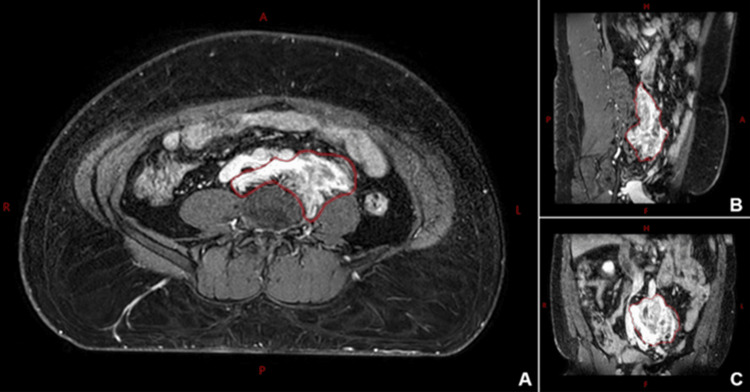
Retroperitoneal ganglioneuroma mass on a post-contrast T1-weighted MRI in axial (A), sagittal (B), and coronal (C) views. The drawn gross tumor volume (GTV) is shown in red. MRI: magnetic resonance imaging
